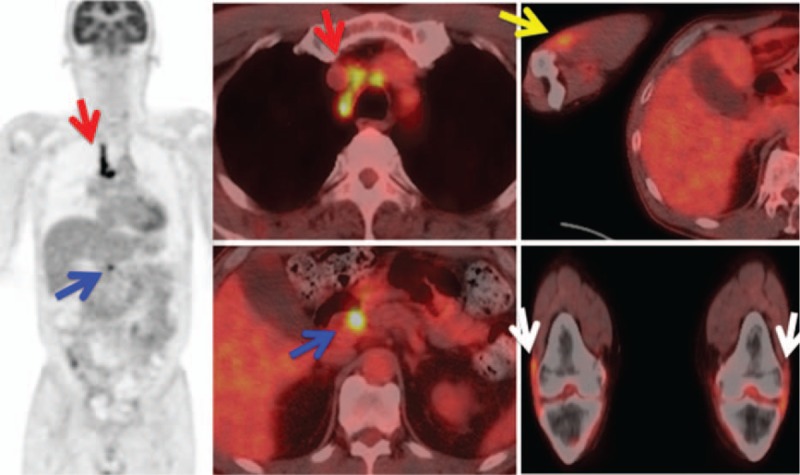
FDG–PET/CT showing moderate uptake by mediastinal (red arrow) and abdominal (blue arrow) lymph nodes, and around the right elbow (yellow arrow) and bilateral knee joints (white arrow).
